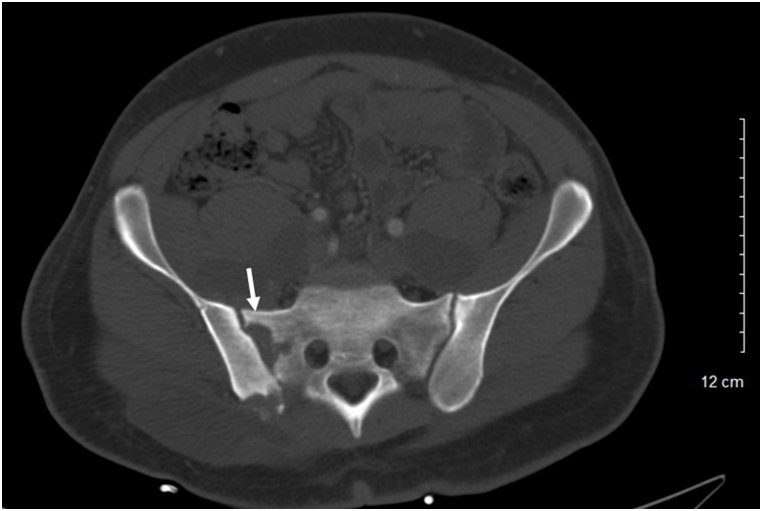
CT radiography of the chest, abdomen, pelvis, head, spine, showing diffuse fluid collections involving the soft tissues and muscles of the abdomen and pelvis, along with focal lytic lesion in the right temporal bone with adjacent focal meningeal thickening, as well as lytic lesions on iliac and sacroiliac regions of the spine.
